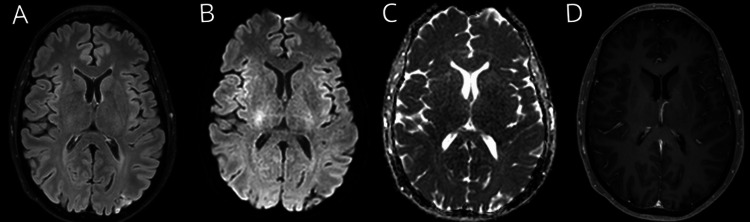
3T Brain MRI, four months later. (A) FLAIR sequence shows near-complete resolution of the left-predominant parieto-occipital hyperintensity.
